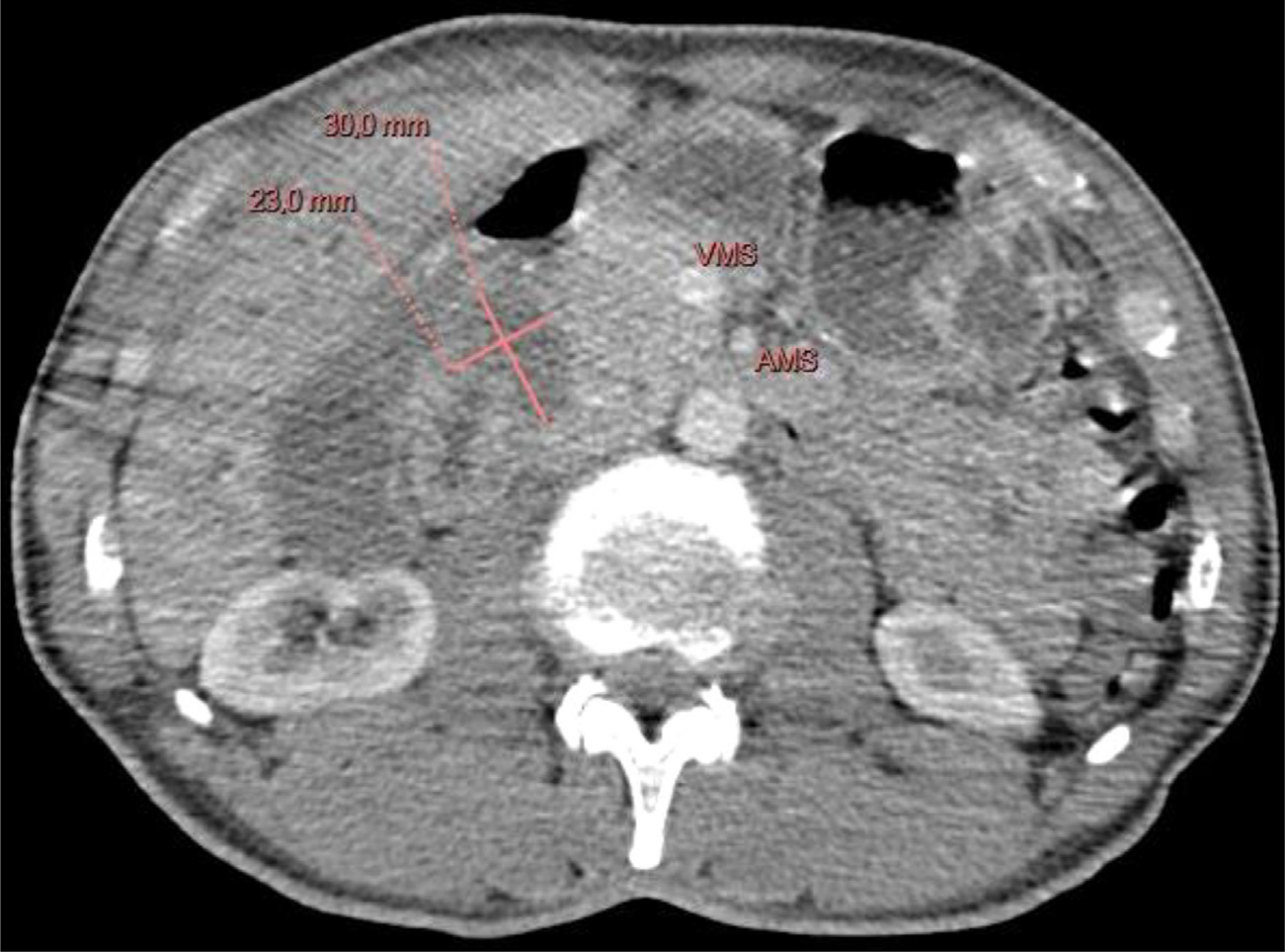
CT scan of the first case showing a pancreatic mass in the pancreatic head with no vascular invasion.
